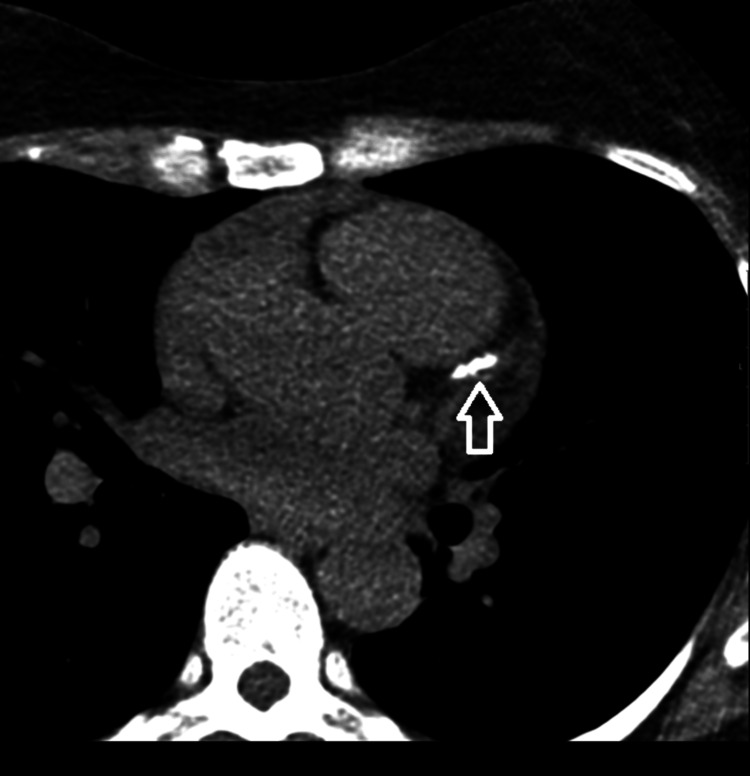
The CT coronary angiogram demonstrates coronary calcification, as indicated by the white arrow.
